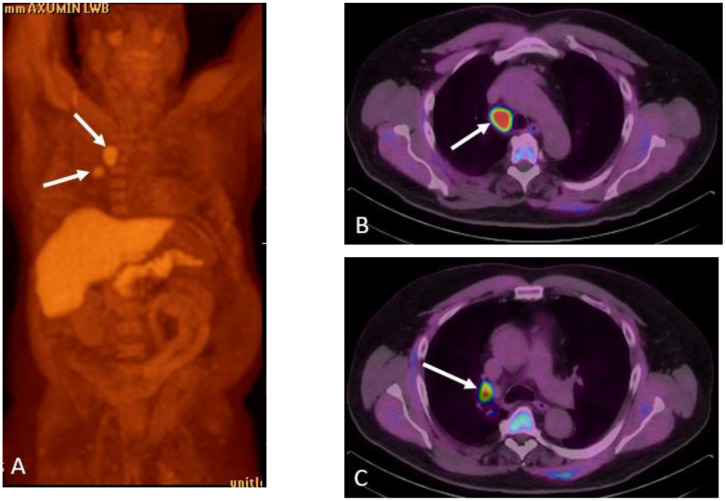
Sixty-seven-year-old man with history of prostate cancer status post-prostate, and salvage radiotherapy with rising PSA. Axumin MIP (A) and fused PET/CT (B,C) demonstrated right lower paratracheal and hilar lymphadenopathy (arrows) concerning for metastases from prostate cancer or a secondary lung primary. Endobronchial ultrasound fine needle aspiration demonstrated adenocarcinoma consistent with prostate primary cancer. Patient was treated with androgen deprivation therapy and localized radiotherapy with nadir to undetectable PSA.
